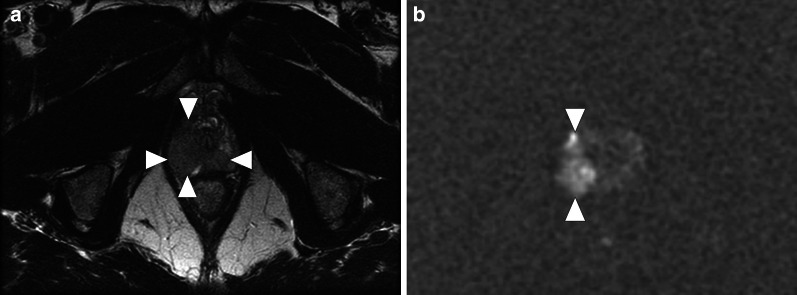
Granulomatous prostatitis after BCG therapy. A 62-year-old man with a history of bladder cancer treated with cystoscopic surgery and intravesical BCG therapy now presenting with deep pelvic pain and difficulty with urination. Axial T2-weighted image a of the prostate demonstrates a large hypointense lesion (arrowheads) on the right lateral and posterior peripheral zone of the prostatic apex. Axial diffusion-weighted image b (b = 2000s/mm^2^; 3 T) demonstrates pronounced diffusion restriction (arrowheads) concerning for prostate cancer. Fusion-guided transrectal biopsy confirmed tuberculous prostatitis with no evidence of malignancy
